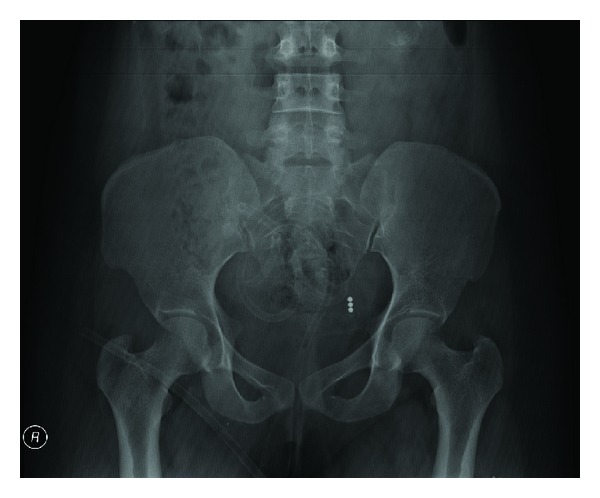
Straight X-ray PA view of the abdomen and pelvis showing the coiled foreign body occupying the pelvis and lower abdomen with three radiolucent beads at one end.
